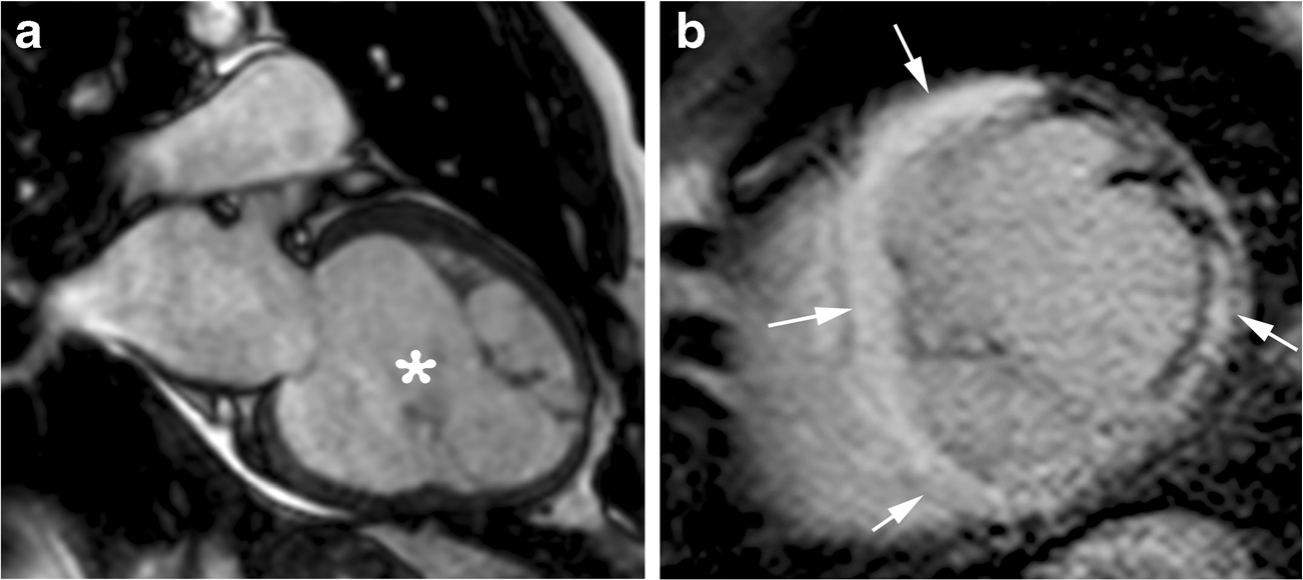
End-stage HCM with dilated form. 69-year-old man with long-standing asymmetric septal HCM who presented with dyspnea at rest and congestive heart failure. a, Two-chamber SSFP cine MR image shows regression of wall thickness, increase in left ventricular cavity dimensions (asterisk) and a reduced ejection-fraction of 33%. b, Short-axis inversion-recovery image showing transmural (arrows) delayed enhancement involving more than 25% of myocardial mass
